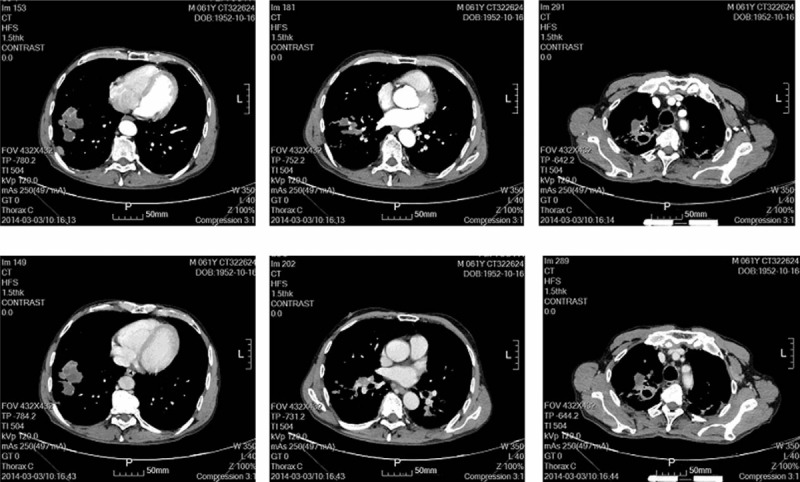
An enhanced CT scan showing that the enhancement of right lower lung fields was not evident.
